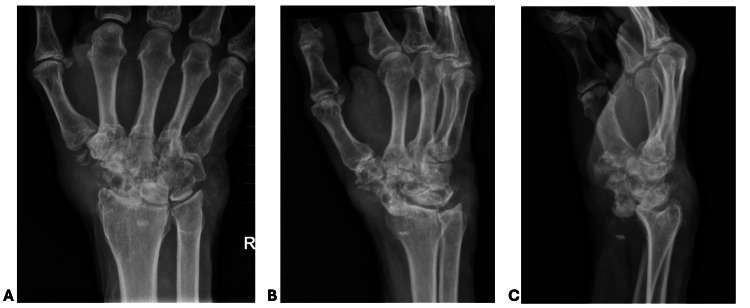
Injury radiographs of the right wrist AP: anteroposterior AP (A) and oblique X-ray (B) of the right wrist with extensive erosive changes throughout the carpus and soft tissue swelling surrounding the wrist. Lateral x-ray (C) demonstrating a frank volar scaphoid dislocation. Metacarpophalangeal (MCP) joints appear well-preserved in all projections
